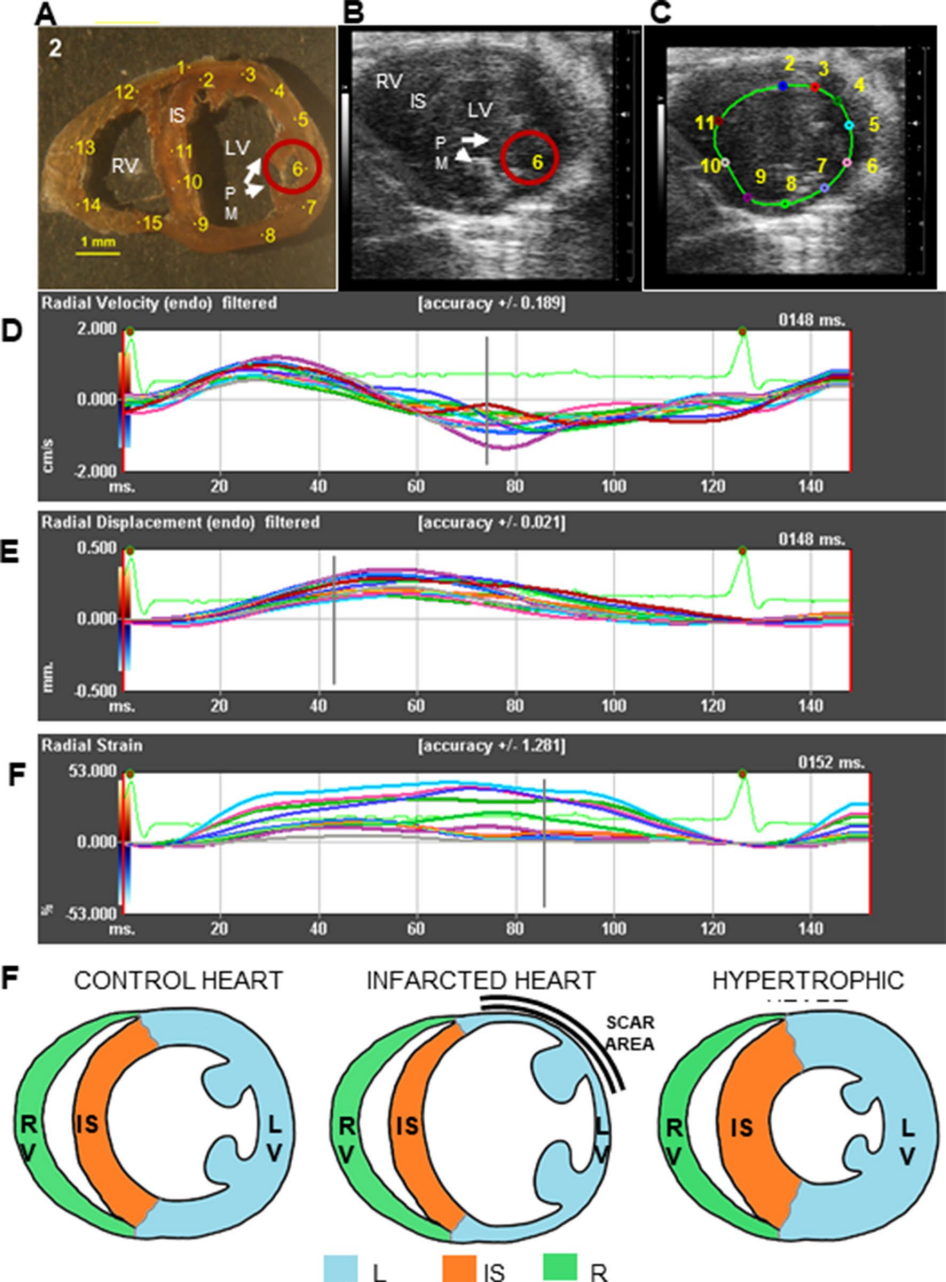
Speckle tracking echocardiography and corresponding segments. (A) Myocardial points analysed by mBS in a representative control heart. (B, C) Short axis echocardiography image (B) with the selected points to be tracked (C). (D–F) Representative radial velocity (D), radial displacement (E) and radial strain (F) measured along time for each selected point. (G) Heart segments analysed in the study. *RV* right ventricle, *IS* interventricular septum, *LV* left ventricle, *ms* milliseconds.
